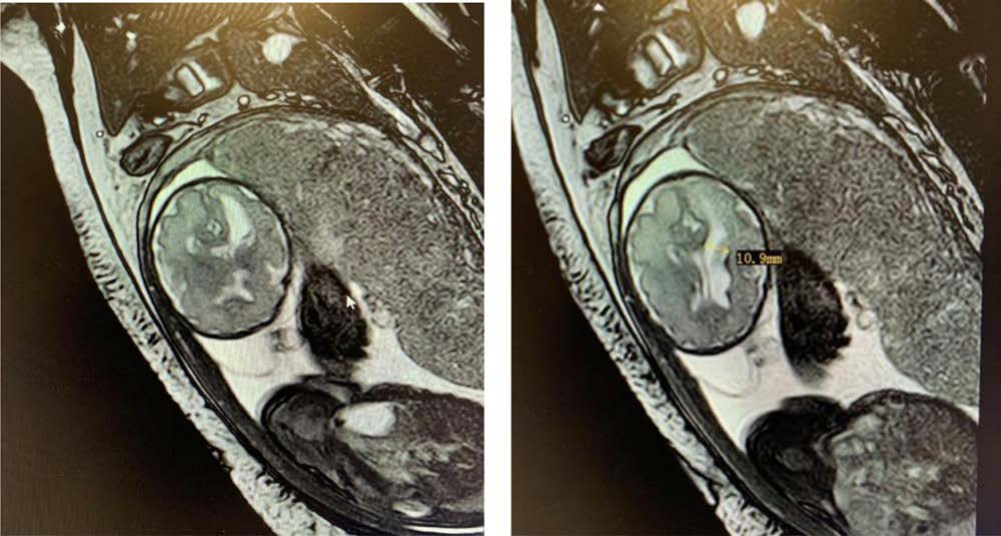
Magnetic resonance image of intracranial hemorrhagic lesions of twin B at 30 wk.
